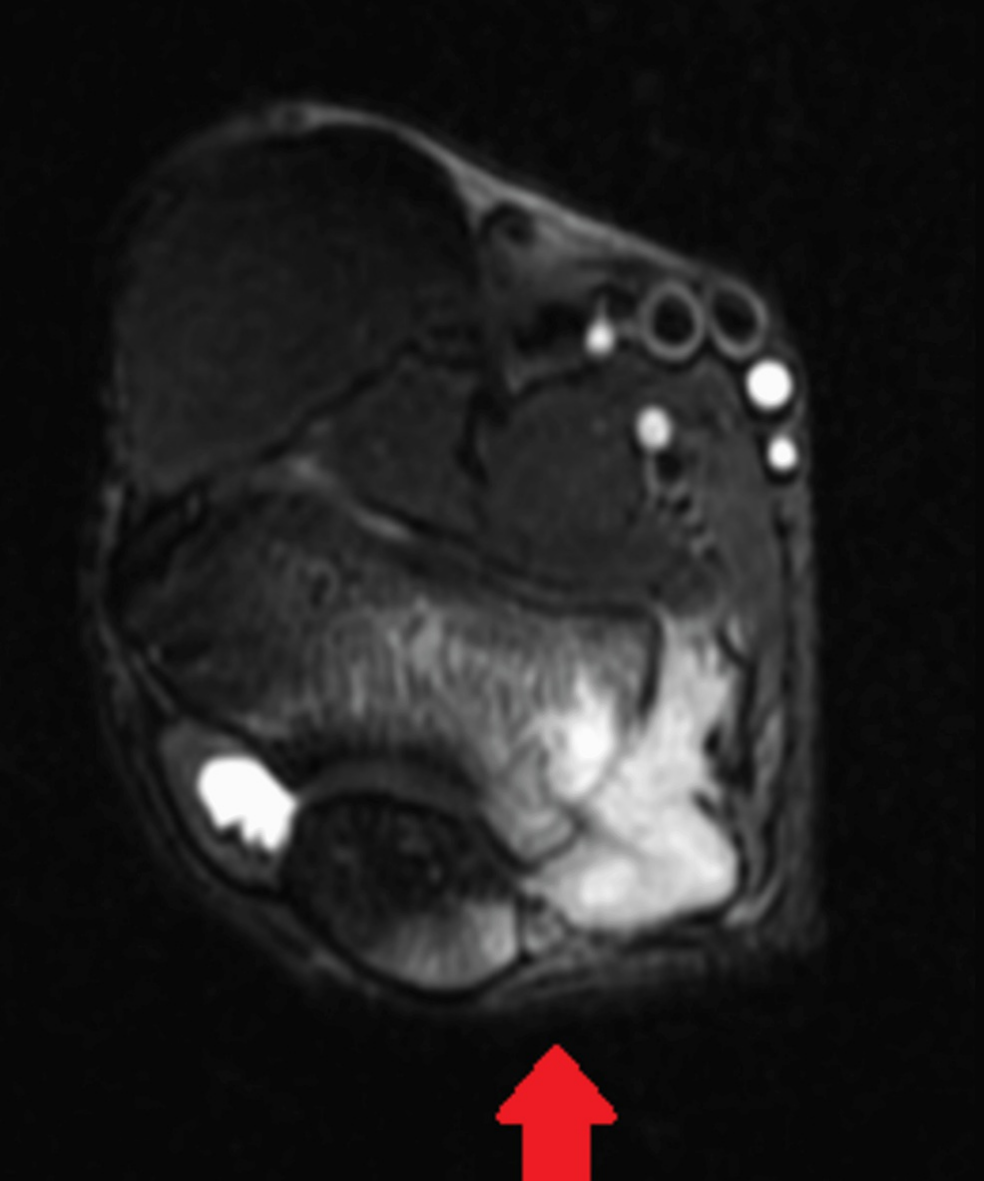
MRI of the left elbow joint Arrow showing a T2-weighted image with involvement of the medial epicondyle of the left humerus MRI: magnetic resonance imaging
